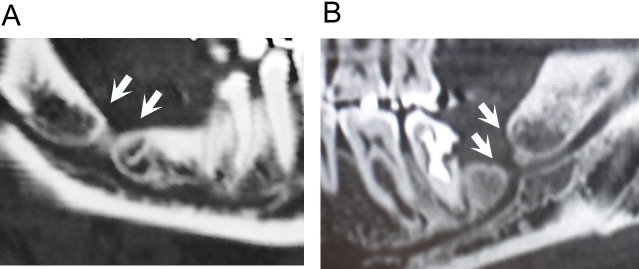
Sclerotic changes in alveolar bone after IAN injury. CT, X-ray and photographic images from patients of a post-extraction tooth cavity. (A) A thirty-five-year-old woman reported dysesthesia for a long period after the tooth extraction. Sclerotic changes were observed in the periphery of the alveolar bone (arrows). (B) A 46-year-old woman experienced dysesthesia, which increased in relation to the deformity of the mandibular canal. In both of these patients, the masses of traumatic neuroma located in the nerve lesion were detected later by surgical observation.
